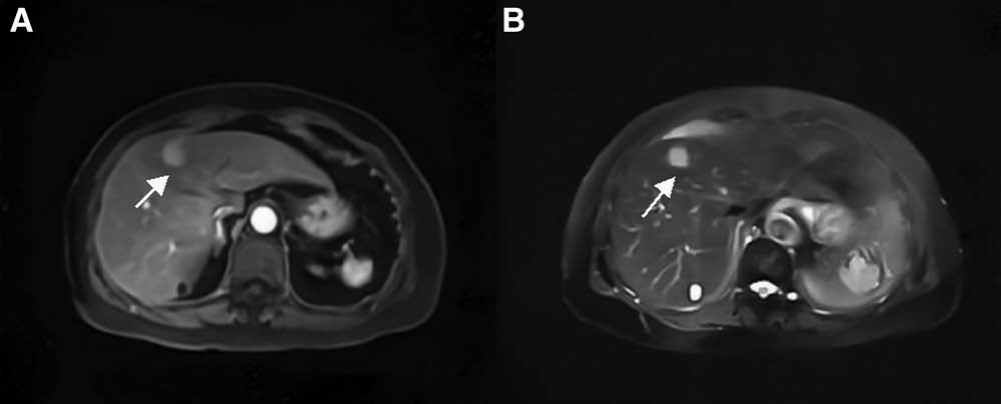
Preoperative MRI. (A) Dynamic enhancement of the mass showed low signal in T1-weighted images. (B) T2-weighted images and diffusion-weighted images showed high signal areas in the lesion. MRI = magnetic resonance image.
